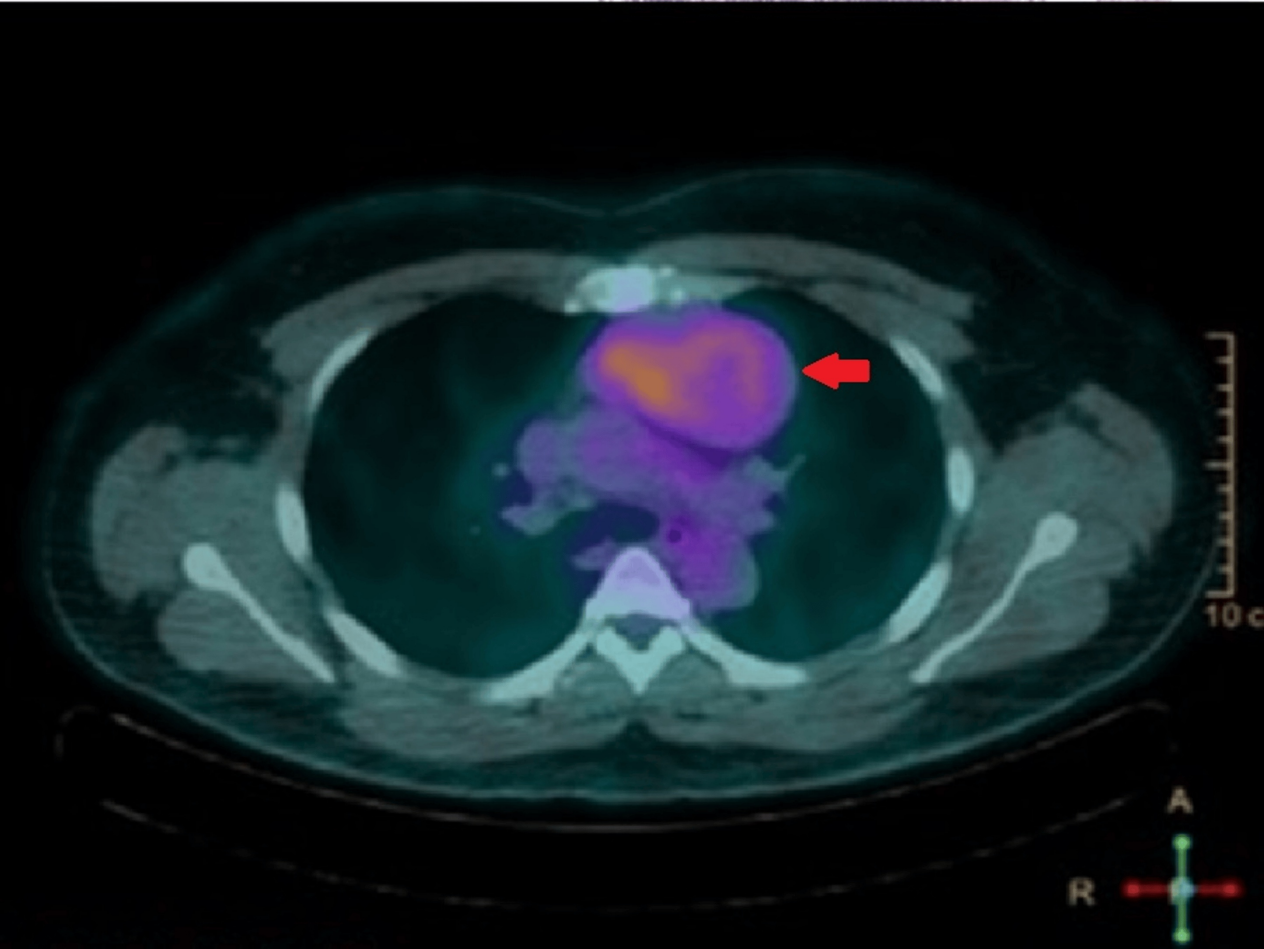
Positron emission tomography (PET): The lesion in the anterior mediastinum (red arrow) demonstrates heterogeneous increased uptake of 18F-FDG, with an SUVmax of 3.81.
